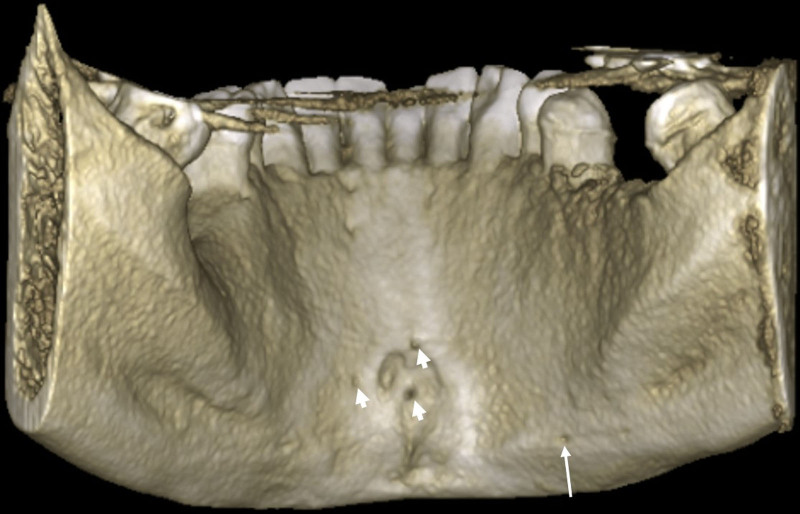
3D reconstructed CBCT image. Median lingual foramina (short arrows), lateral lingual foramen (long arrow). 3D = three-dimensional, CBCT = cone beam computed tomography.
